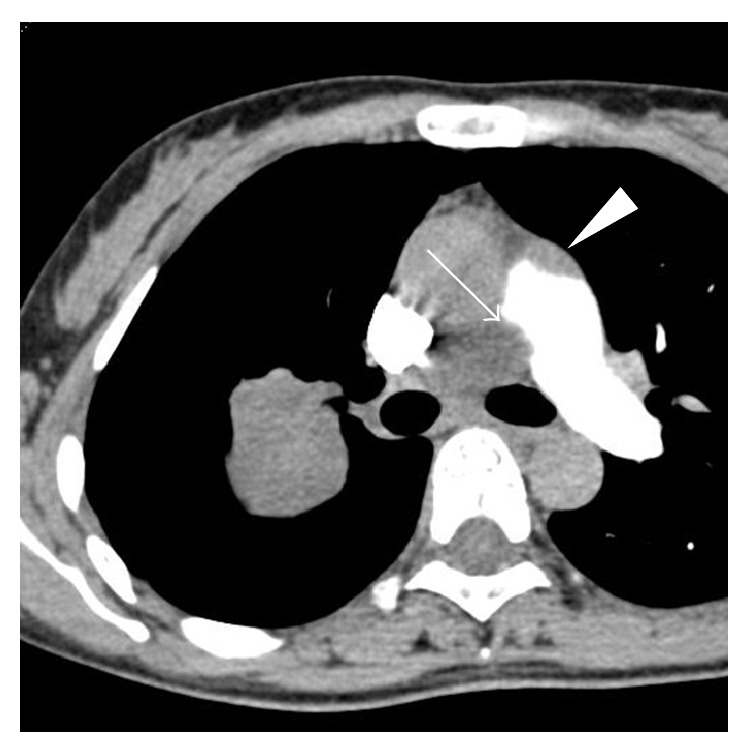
Contrast-enhanced CT showing obstruction of the right pulmonary artery (arrow). A skip lesion was also suspected in the periphery of the pulmonary artery trunk (arrowhead).
